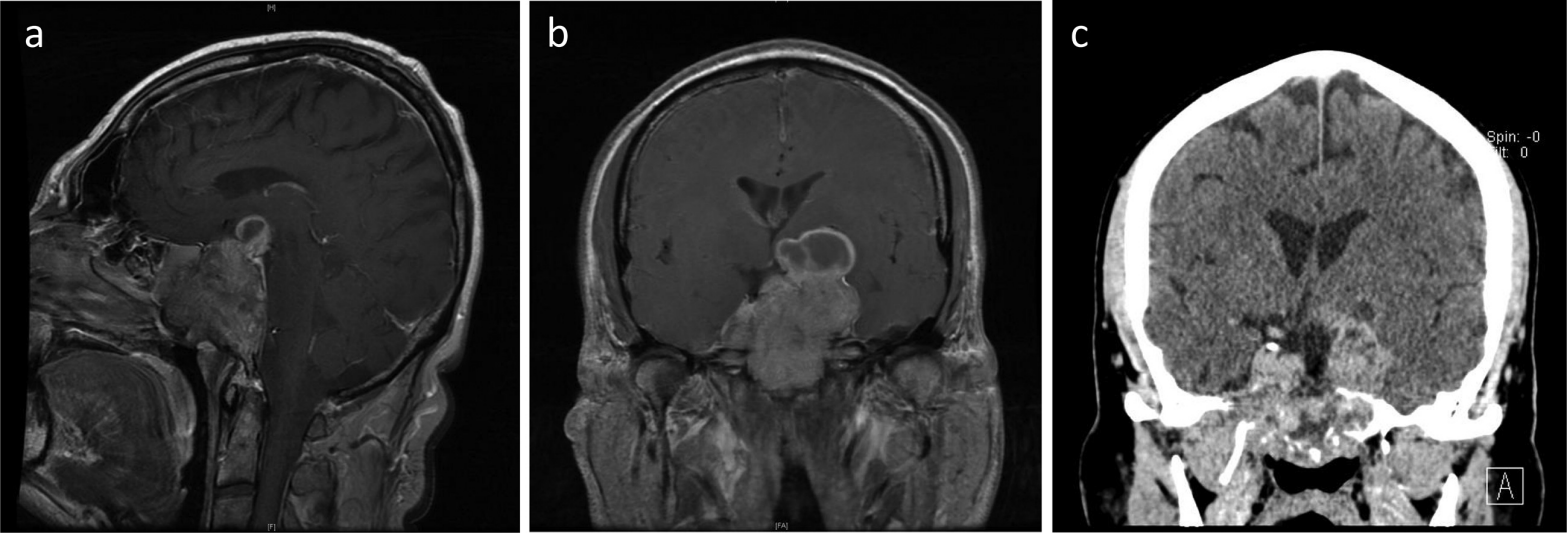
(a) Preoperative *T*_1_ weighted sagittal and (b) coronal MRI scan with contrast, and (c) CT with contrast demonstrated the large pituitary tumour with suprasellar extension, inferior invasion and destruction of the skull base.
